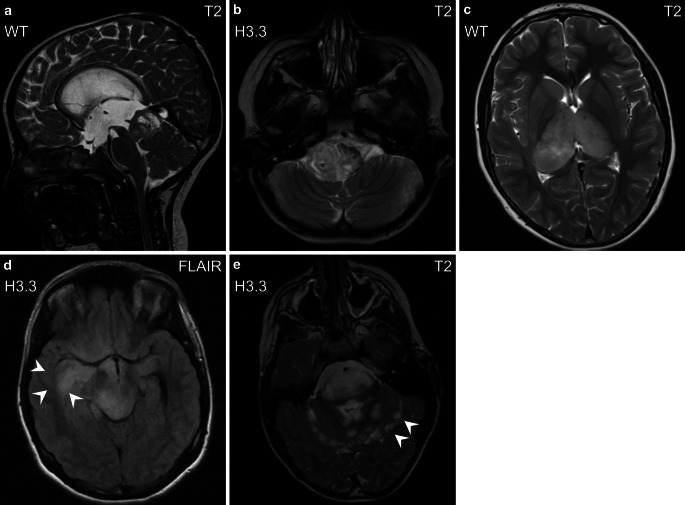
Other localizations of H3 K27M mutant and WT tumors in the midbrain/tectum (a, sagittal images) and medulla oblongata (b, axial images). Note that bithalamic gliomas (c, axial images) typically harbor epidermal growth factor receptor (EGFR) mutation rather than histone H3 mutation [29]. Rare MRI phenotypes with diffuse infiltrative growth (*arrowheads* in d, axial images) and with leptomeningeal spread at diagnosis (*arrowheads* in e, axial images)
